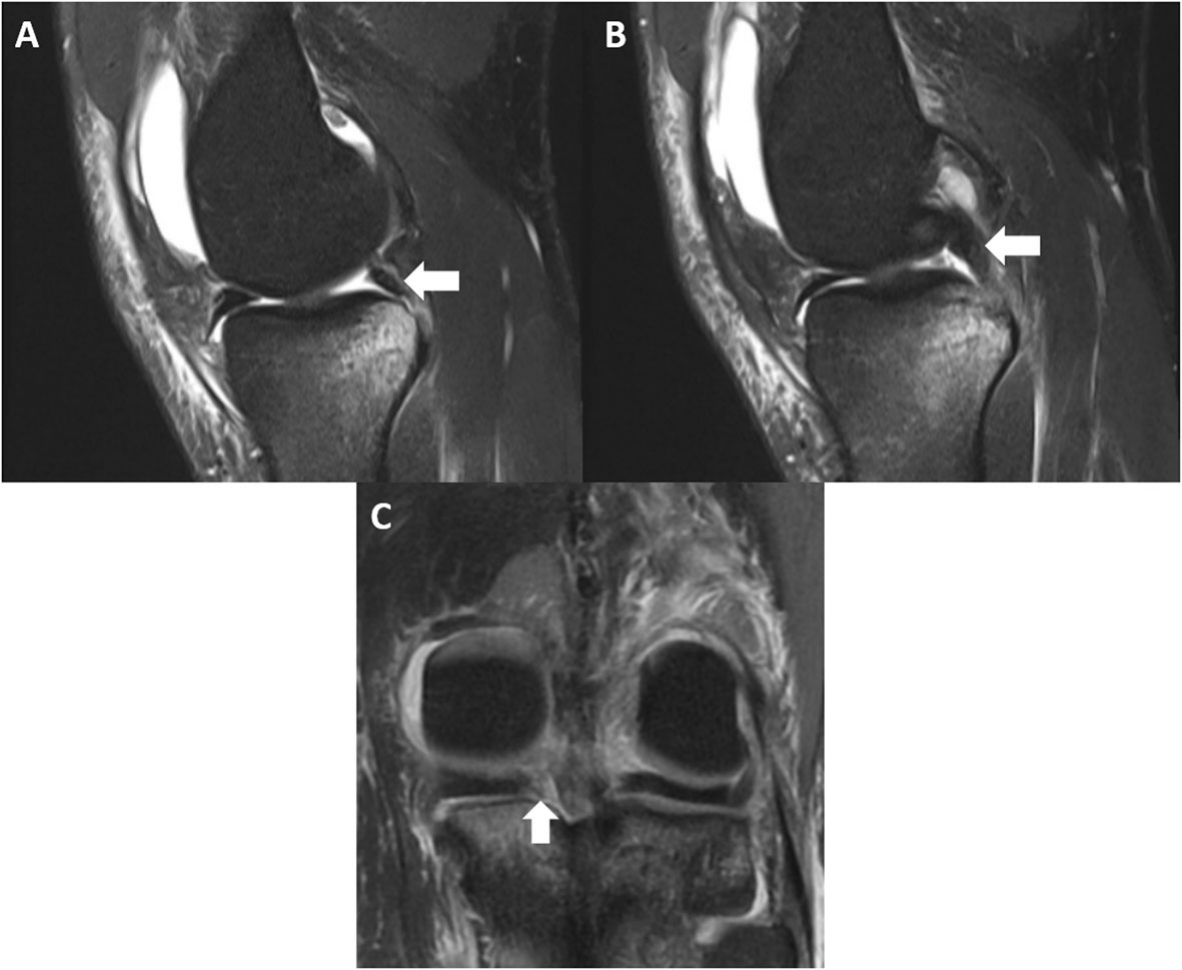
A magnetic resonance imaging of a medial meniscus root tear in conjunction with an anterior cruciate ligament tear. The white arrows point to the meniscus root as it enters its insertion on the tibia. On the top images (a and b), there is fluid underneath the root with no clear attachment to the tibia. The bottom image (c) demonstrates no clear attachment of the root to the tibia
